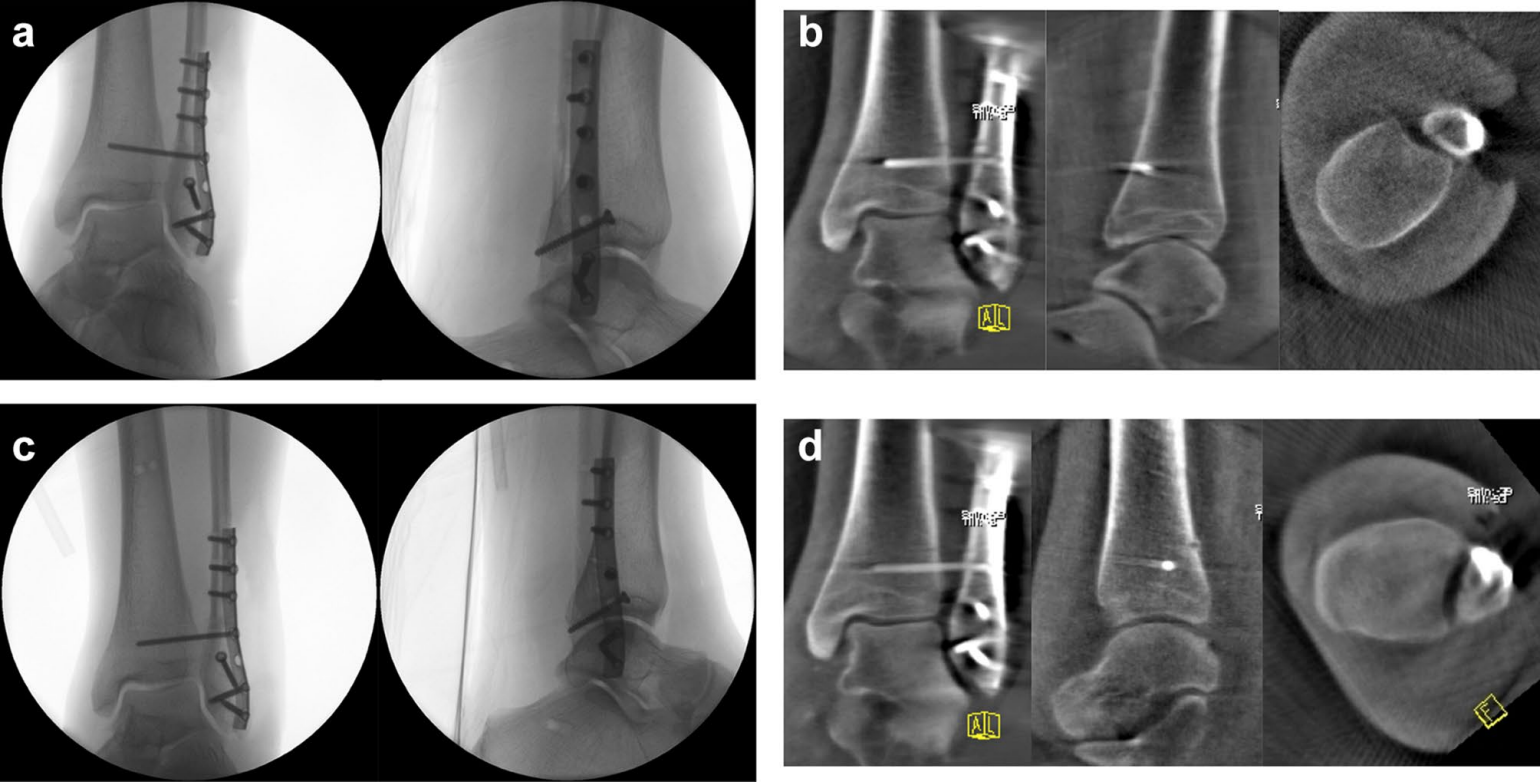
a–d Fluoroscopic images AP and lateral of the ankle after open reduction and internal fixation. In conventional fluoroscopy, anatomic reduction can be assumed (a). The cone beam CT images in the three standard planes demonstrate a posterior translation of the fibula (b). After correction, conventional fluoroscopy again reveals an anatomic reduction (c). The cone beam CT images now displays an anatomic reduction of the fibula in the fibular notch
